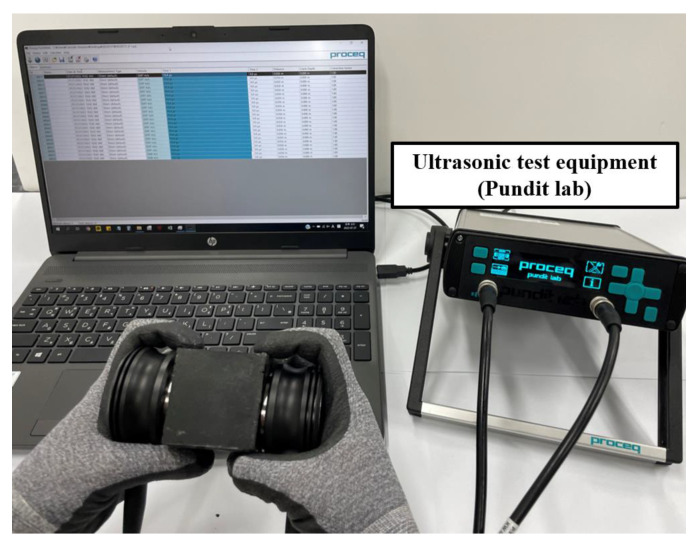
Ultrasonic non-destructive test set-up.
